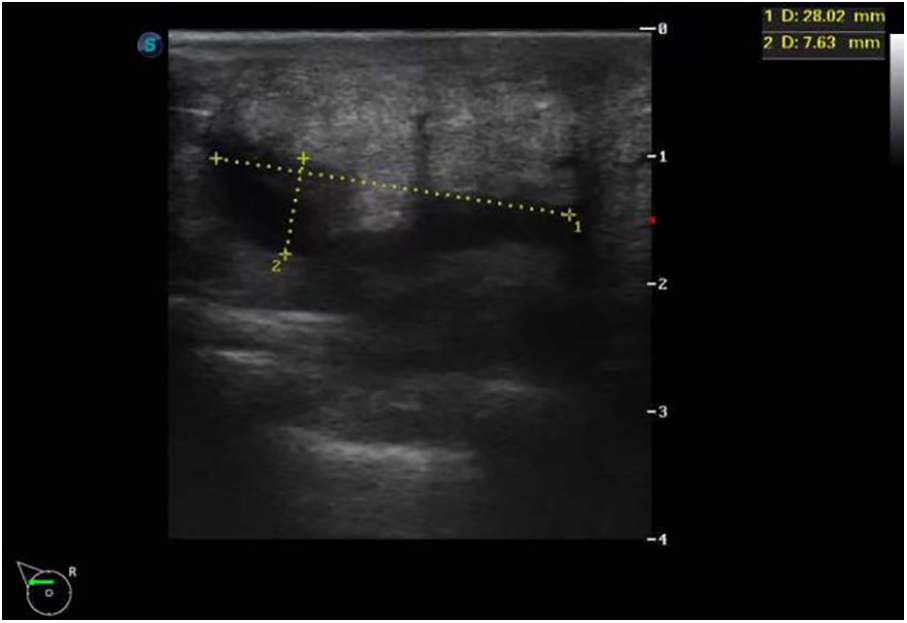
Color Doppler ultrasound indicated a small amount of fluid accumulated in the surgical area.
